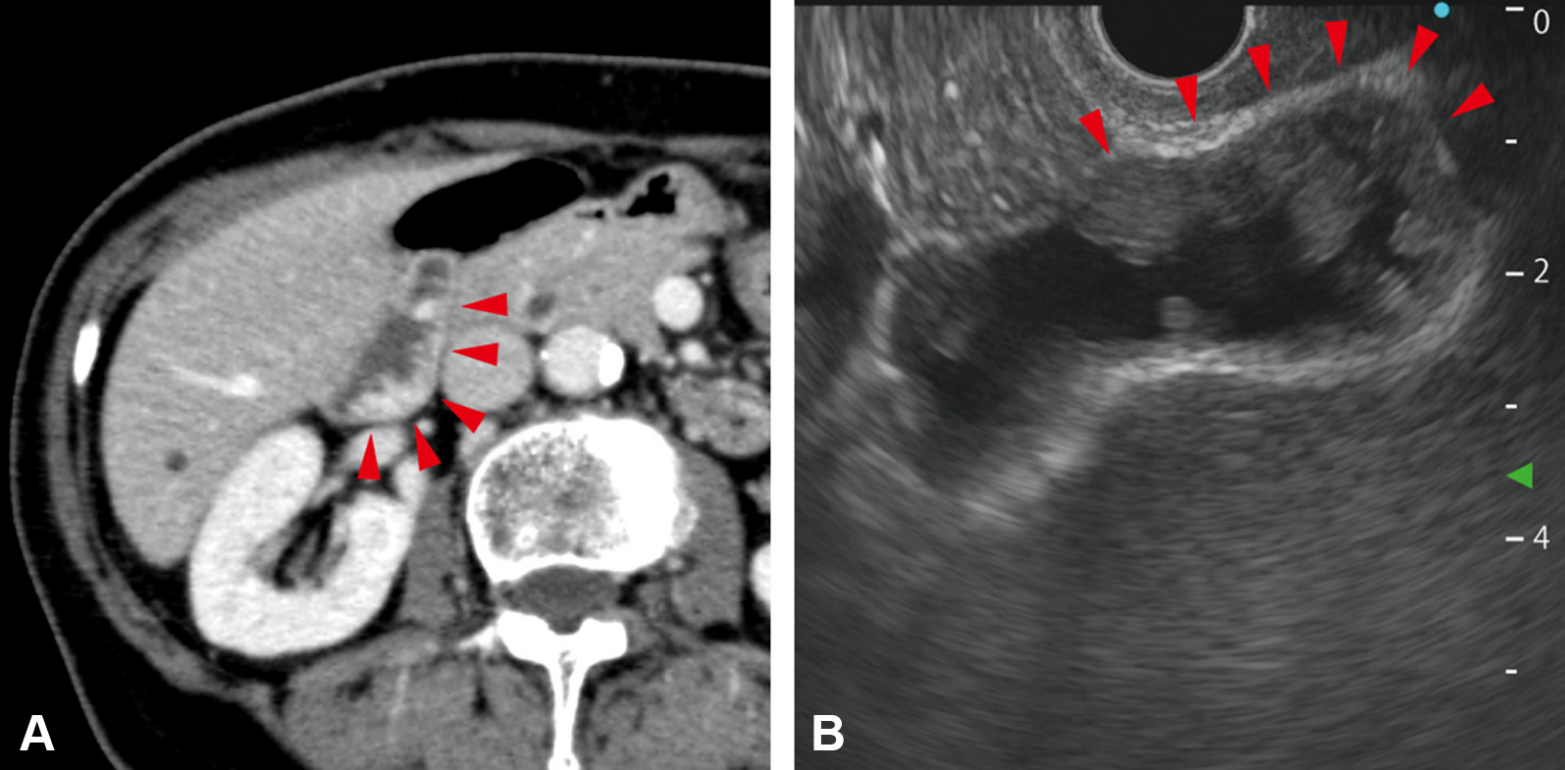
Images of the gallbladder lesion. A, Contrast-enhanced CT revealed that there was irregular thickening of the gallbladder wall (*red arrows*), although extramural invasion was not evident. B, EUS revealed that there were many mobile polyps larger than 10 mm in the gallbladder. Wall thickening also was observed in a part of the gallbladder wall (*red arrows*), but no extramural invasion was detected.
